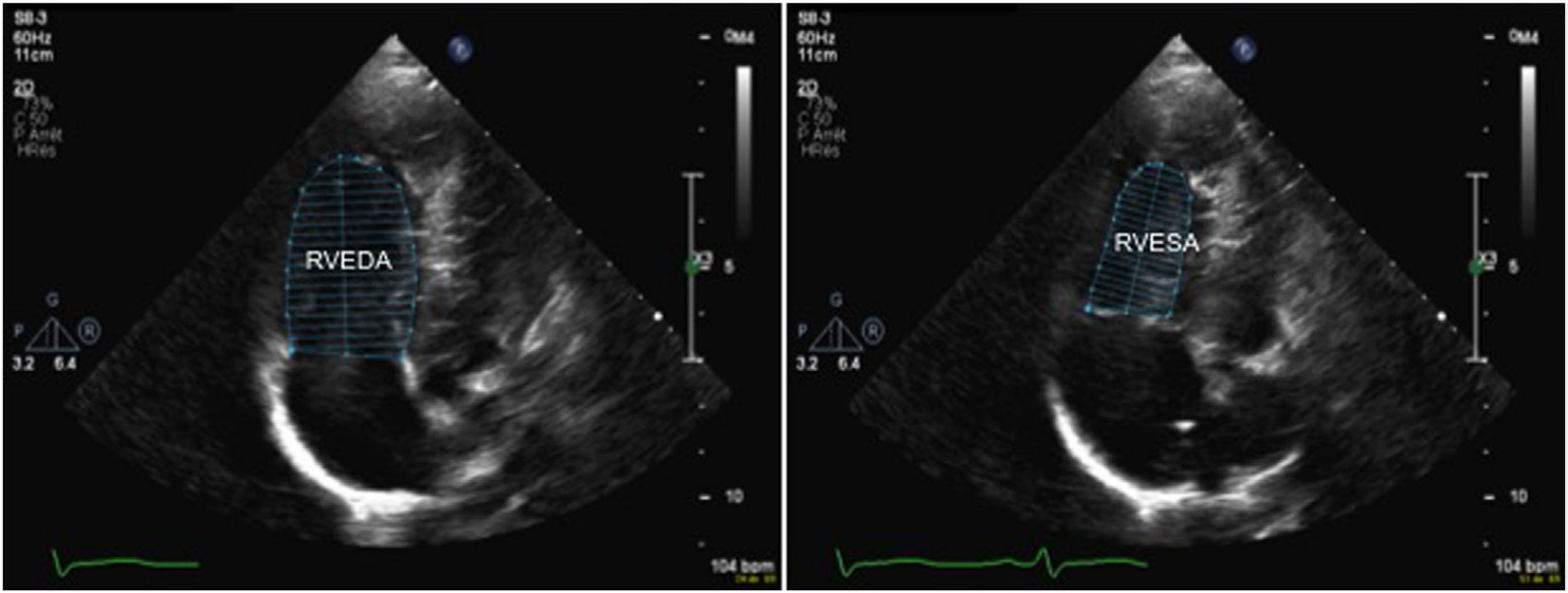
2D echocardiography from an apical four-chamber in a patient with pulmonary hypertension view allowing measurement of the right ventricular fractional area change (FAC) by tracing the endocardial border to obtain the RVEDA and RVESA. A FAC value <35% is indicative of right ventricular systolic dysfunction. Abbreviations: RVEDA, right ventricular end-diastolic area; RVESA, right ventricular end-systolic area.
